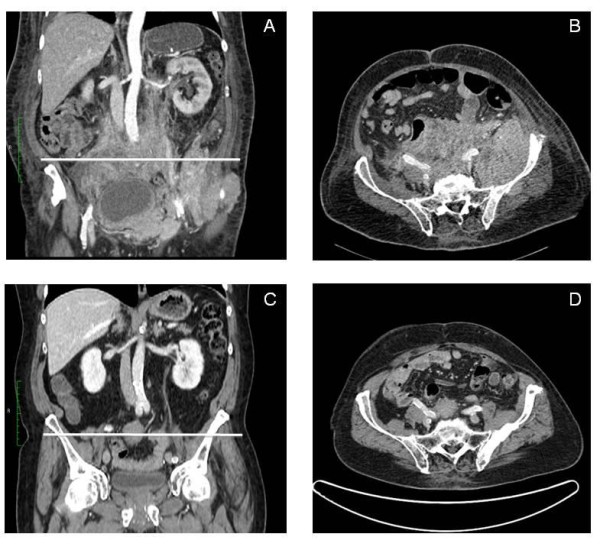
CT of the abdomen before (A, B) and after (C, D) four months of treatment with lenalidomide.
